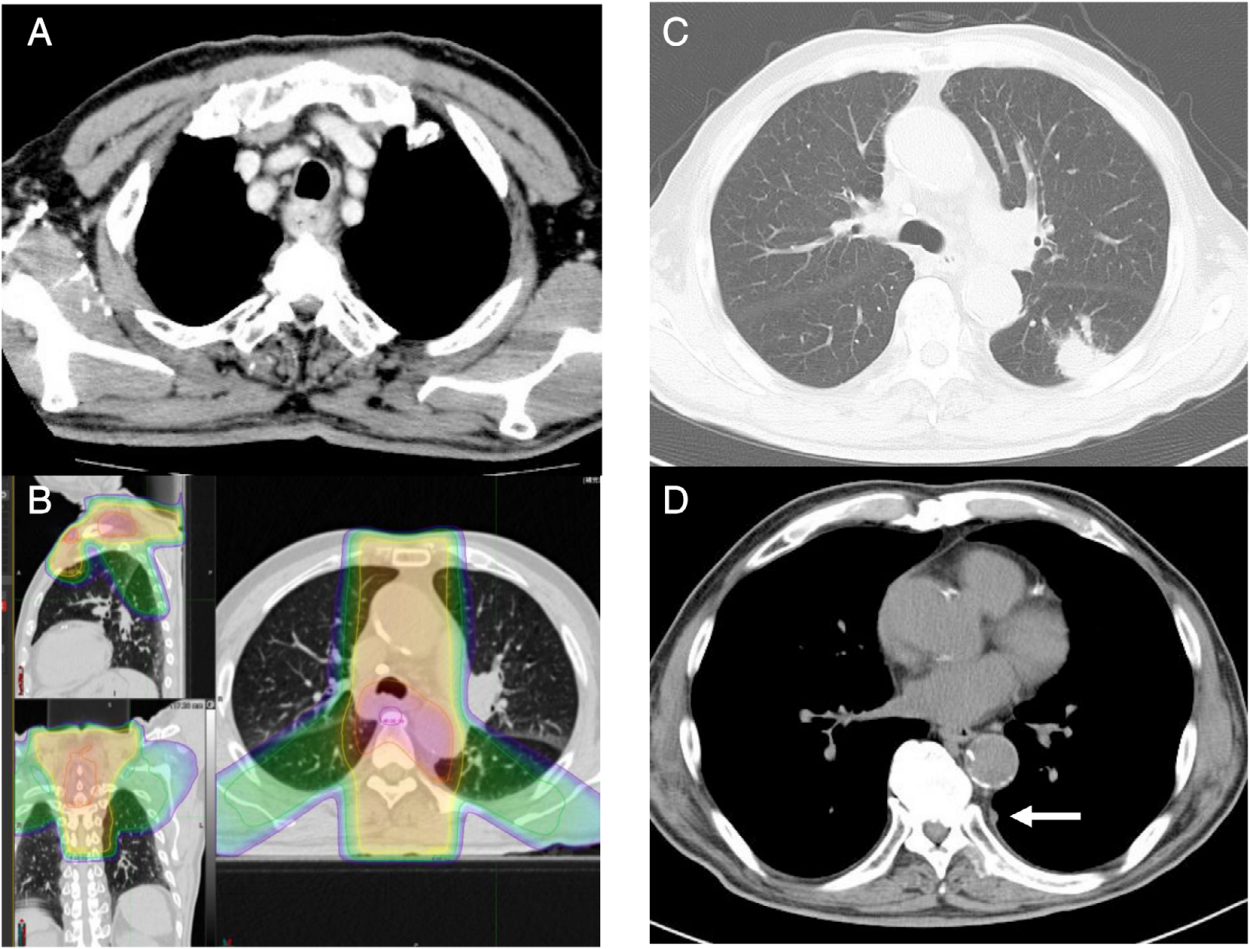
Images of case 2. (A) Thoracic computed tomography showing swelling of the oesophagus at the onset of oesophageal squamous cell carcinoma when the patient was 77 years. (B) Map of radiation dose distribution in radiotherapy for oesophageal squamous cell carcinoma. (C) A nodule seen in the left lower lobe. (D) The arrow indicates a small nodule on the pleura.
